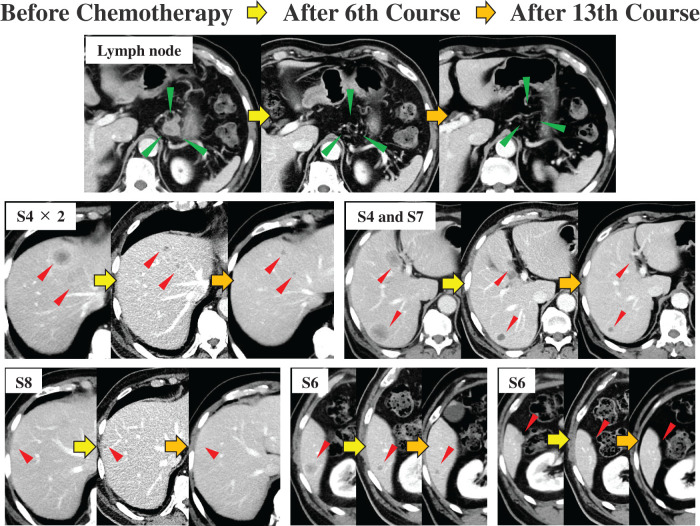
CE-CT findings before, during, and after chemotherapy. Before chemotherapy, a 27 mm mass was observed adjacent to the lesser curvature of the stomach and was identified as a metastatic lymph node (green arrowheads). Multiple liver metastases were also detected (red arrowheads), with three lesions in segment 4 (S4), two in S6, and one lesion each in S7 and S8. After the 6th course of chemotherapy, the metastatic lymph node exhibited marked shrinkage and were almost undetectable. The liver lesions also showed significant shrinkage. The shrinkage of each lesion was maintained even after the 13th course of chemotherapy. All lesions were completely resected during conversion surgery. CE-CT, contrast-enhanced computed tomography
